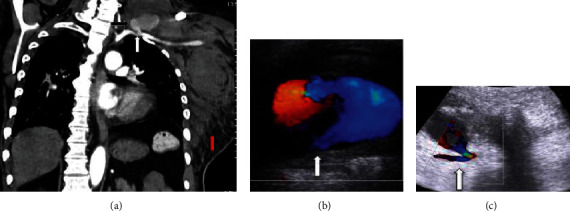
(a) CT coronal section showing the left subclavian artery (white arrow) with pseudoaneurysm (black arrow) and large intra- and extramuscular chest wall and left side hematoma (red arrow). (b, c) Ultrasonography showing a pseudoaneurysm originating from the subclavian artery (white arrow).
